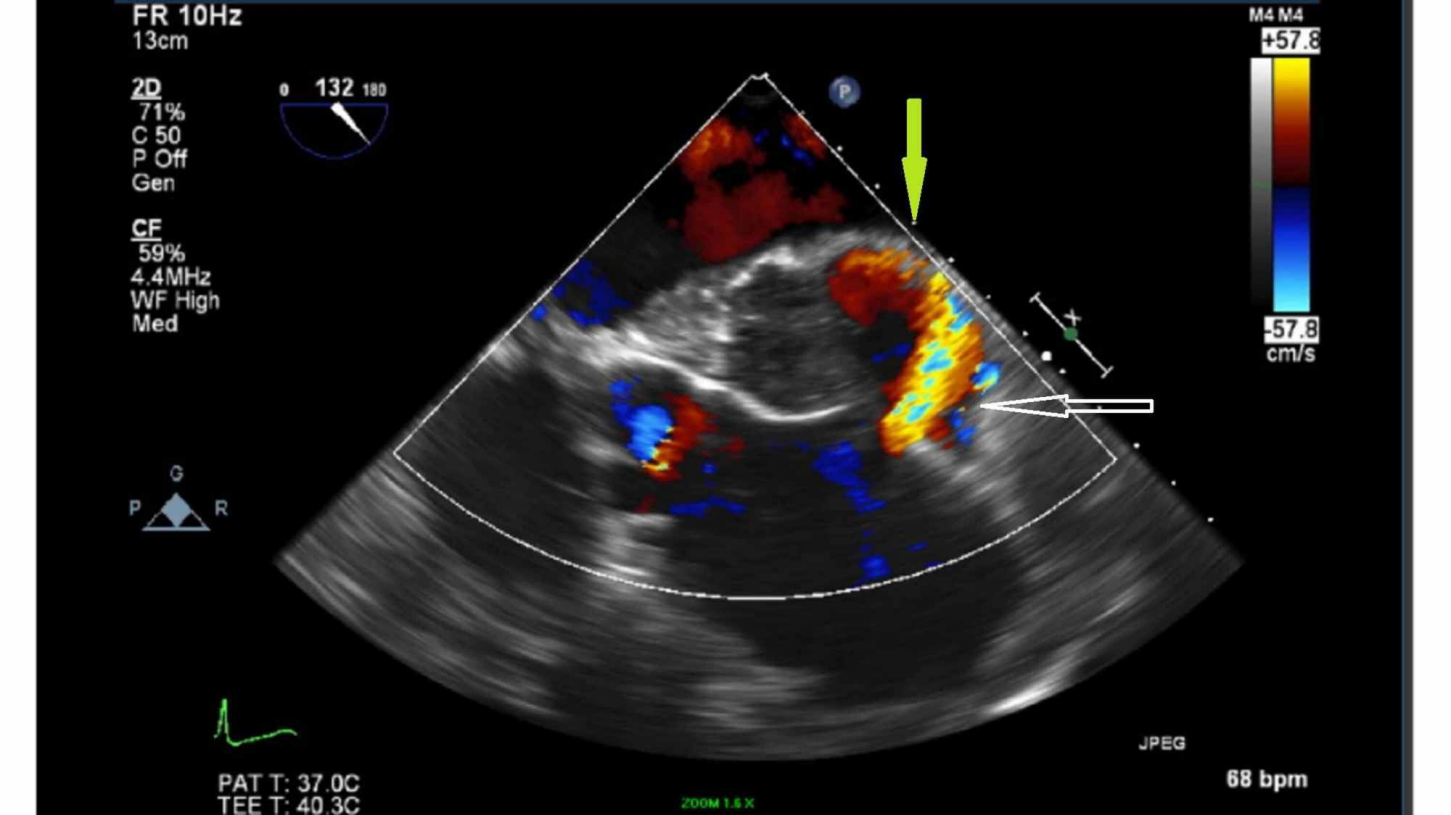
Transesophageal echocardiogram (TEE) TEE shows a proximal ascending aorta dissection, aortic wall tear, blood flow into the transverse pericardial sinus (white arrow), and a hematoma compressing the left atrium (green arrow).
